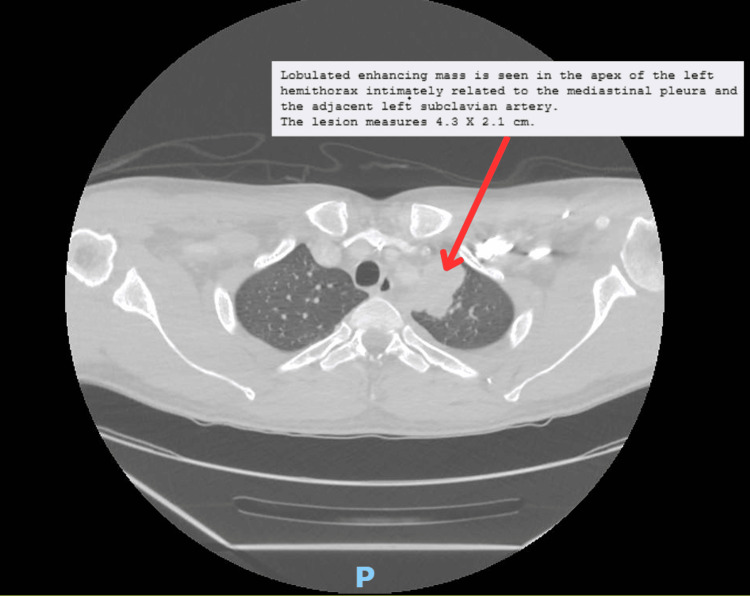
CT of the chest showing a left lung mass.
